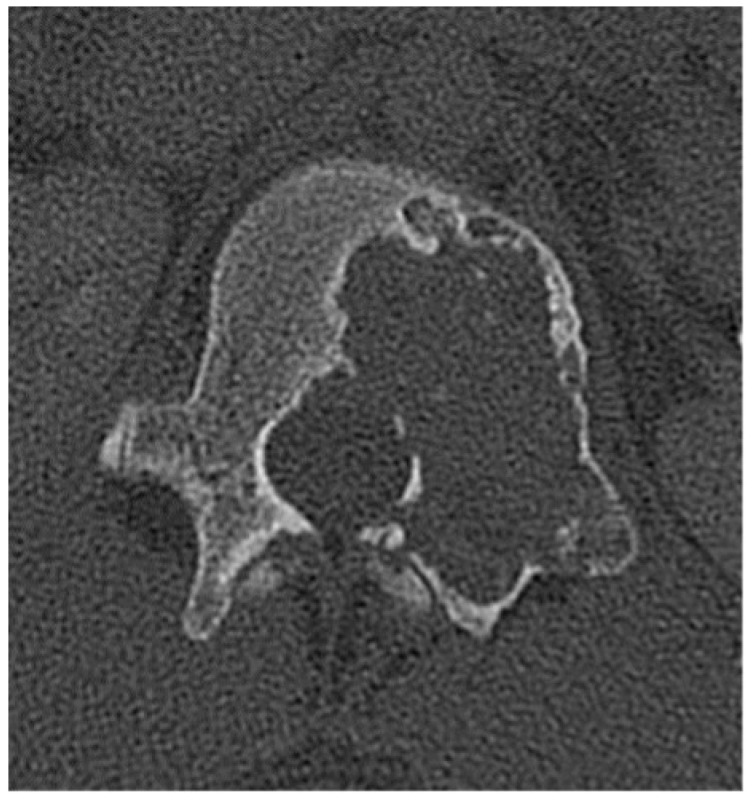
Giant cell tumor. Axial CT bone window demonstrates a giant cell tumor with minimal sclerosis present along the “non-eccentric” border of the lesion which has been described in the literature [74]. Small internally directed bony septations are present.
